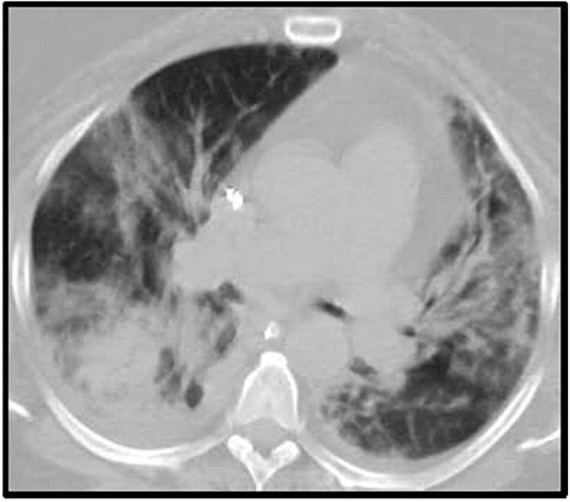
Chest CT after ICU admission. Description: Bilateral multifocal irregular patchy and confluent ground glass and consolidative opacities with associated predominantly bilateral lower lobe irregular reticulations. Small (right) and trace (left) pleural effusion. CT, computerized tomography; ICU, intensive care unit.
